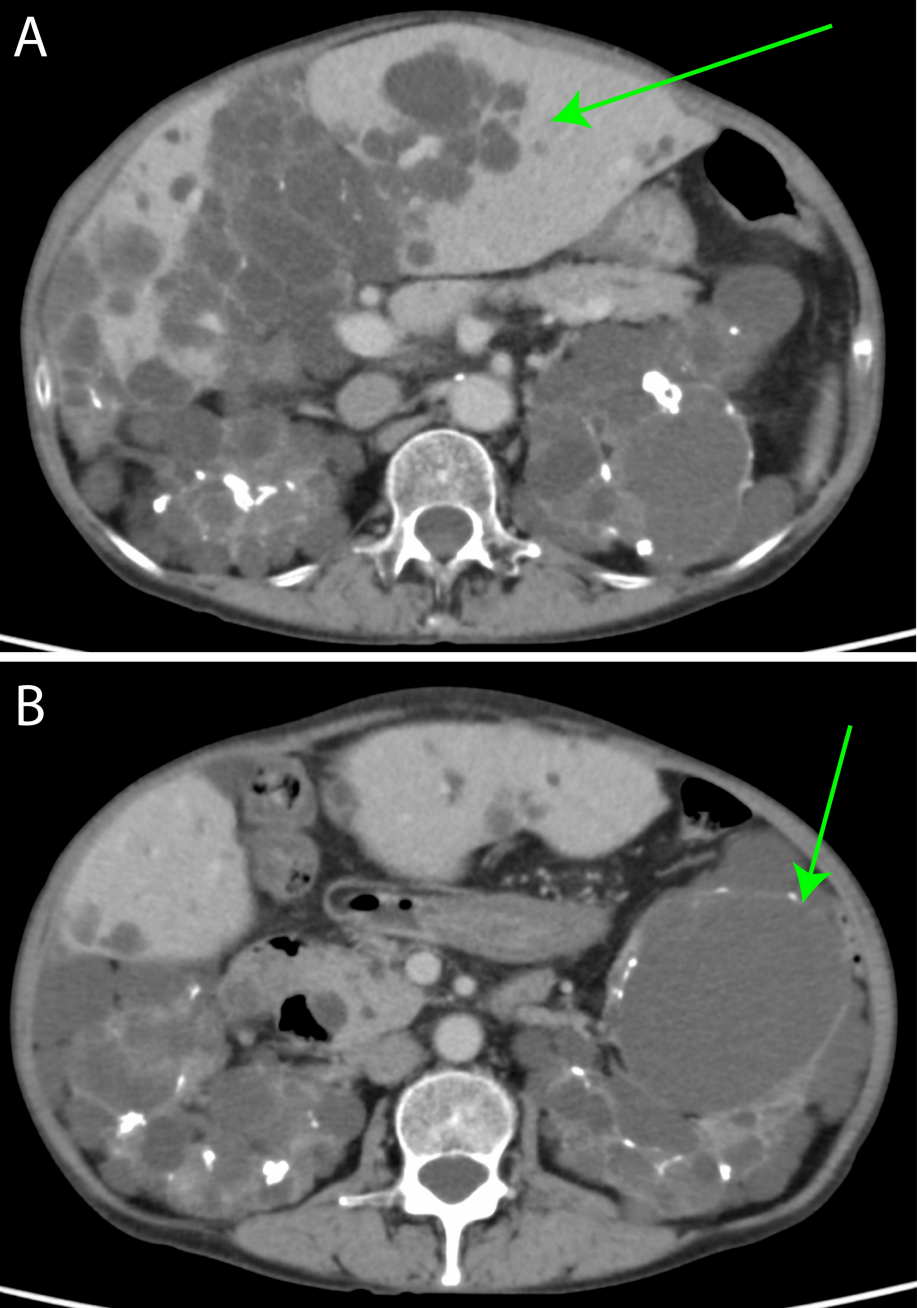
Contrast-enhanced computed tomography at the age of 55 years old. (A) Multiple cysts are observed in the liver and upper pole of the kidney (green arrow). (B) Numerous cysts are observed in the middle part of the kidney (green arrow).
